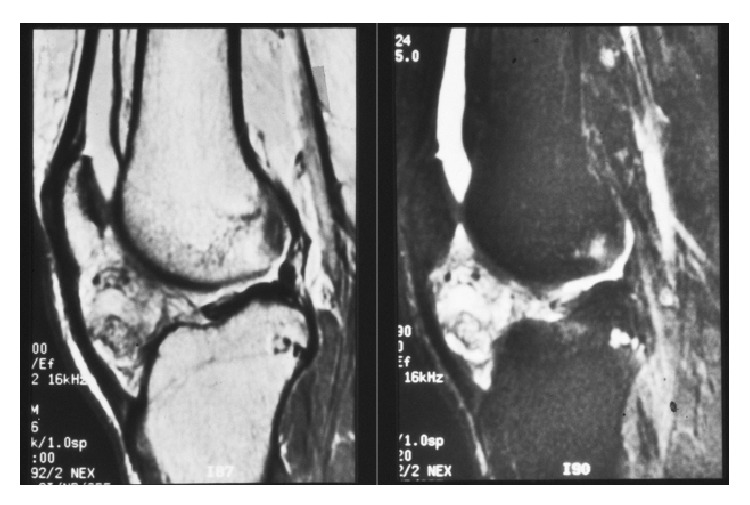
Preoperative MR images, sagittal plane T1- and T2-weighted images, of the knee joint.
